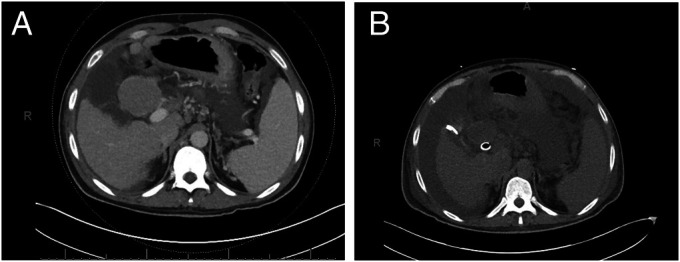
Imaging findings in patient 2: (A) gadolinium-enhanced magnetic resonance imaging showing a new 5.2 × 4.8 cm segment 4 lesion (LI-RADS 5). (B) A triple-phase computed tomography scan performed 1 month after initial diagnosis showed a reduction in size to 4.5 × 3.2 cm, suggestive of autoinfarction.
